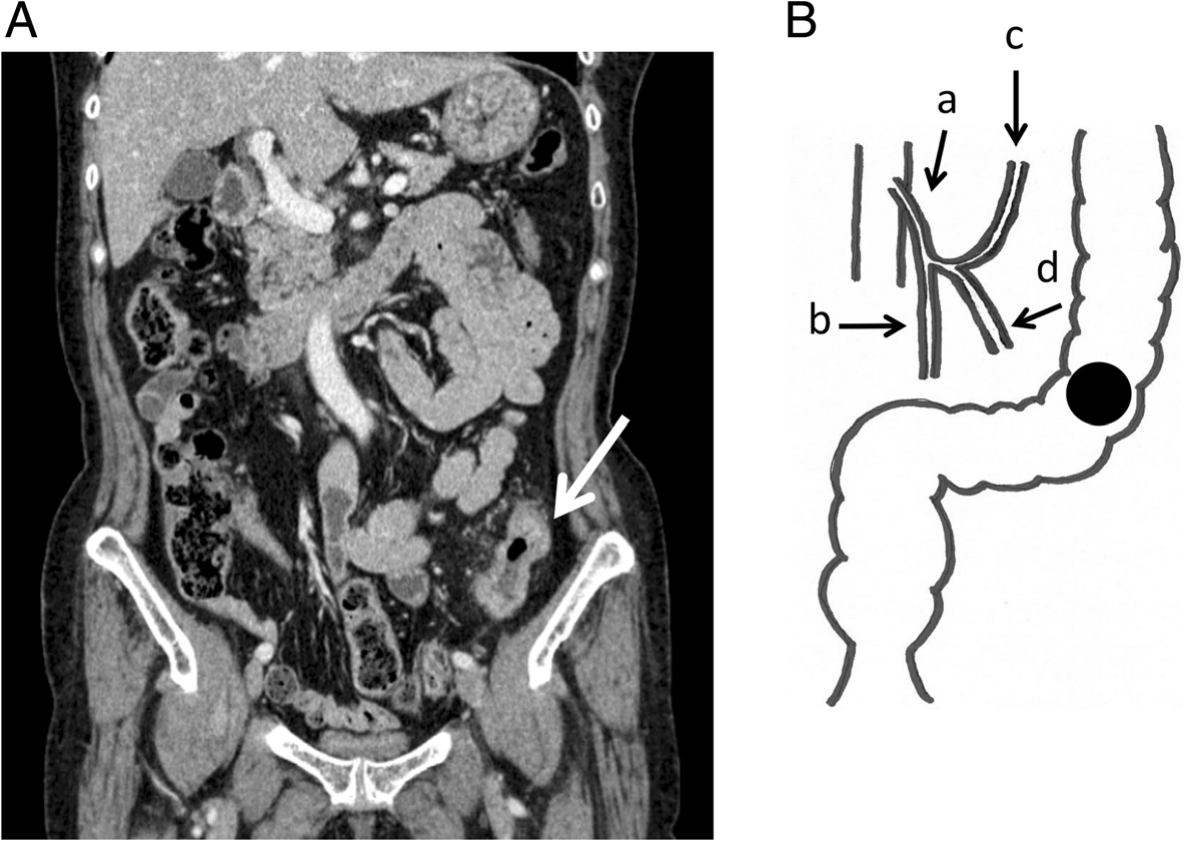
A The abdominal CT scan showed T3 colon cancer near the SD junction. The arrow indicates the tumor location. B Anatomy of this patient. a: inferior mesenteric artery, b: superior rectal artery, c: left colic artery, d: sigmoid artery
